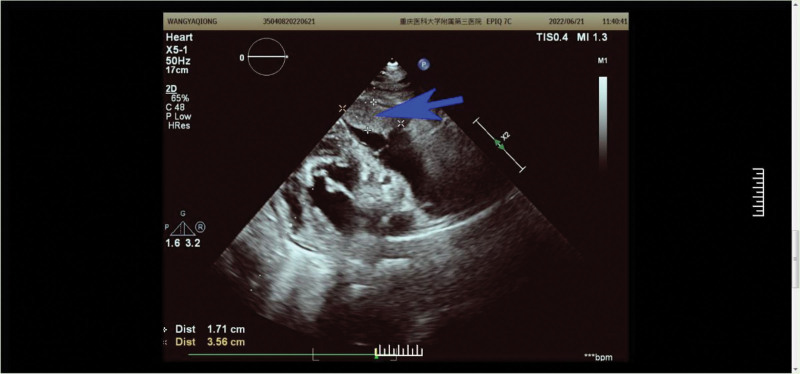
Review color ultrasound on June 21st: the location indicated by the blue arrow indicates a right intraventricular mass (48 × 17 mm).
